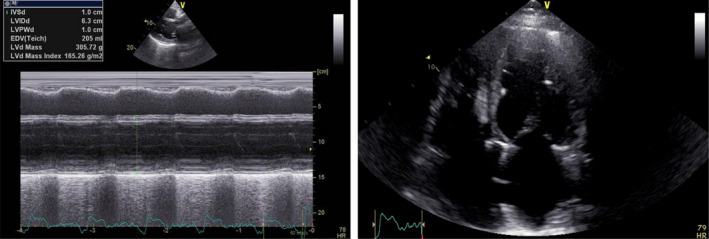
Transthoracic echocardiogram from GA patient. Transthoracic echocardiogram from parasternal long‐axis view (M‐mode) (on the left) and from apical four‐chamber view (on the right) showing a severe dilation of the left ventricle (63 mm) and of the left atrium
